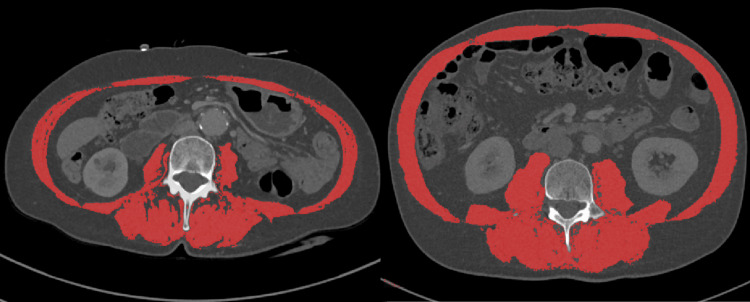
Example of a CT from a sarcopenic patient (left) and a non-sarcopenic patient (right). Highlighted area is the measured skeletal cross sectional area used to calculate the skeletal muscle index
